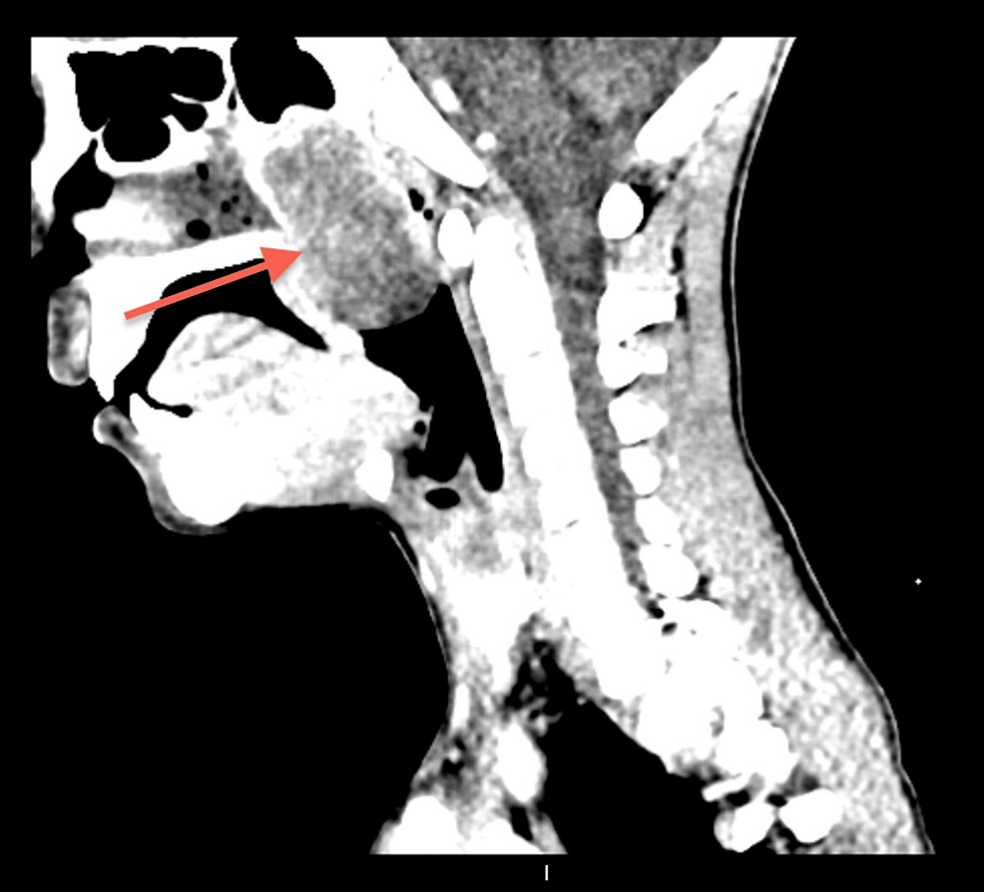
CT Neck Sagittal View CT of the neck showing the adenoid region with a 4.6x2.7 mm cystic lesion remodeling the floor of the sphenoid sinus, displacing the uvula anteriorly and compromising the nasopharyngeal airspace.
